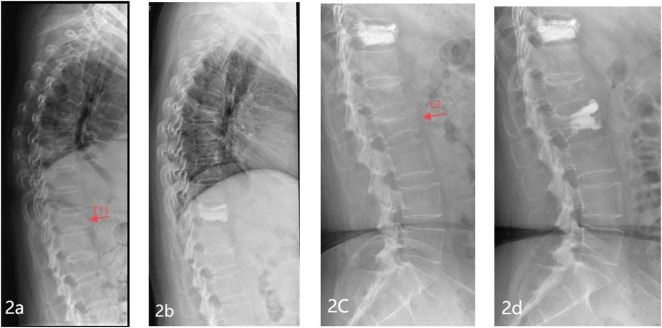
Representative images of a typical case of new vertebral fracture in a patient with OVCF after PVP/PKP. (A) Preoperative lateral X-ray of the thoracic spine showing a T11 vertebral compression fracture; (B) lateral X-ray of the thoracic spine 1 day post-operation showing T11 filled with bone cement; (C) lateral X-ray of the lumbar spine 1 year post-operation showing a new vertebral compression fracture at L2; (D) Lateral X-ray of the lumbar spine 1 day post-operation showing L2 filled with bone cement.
